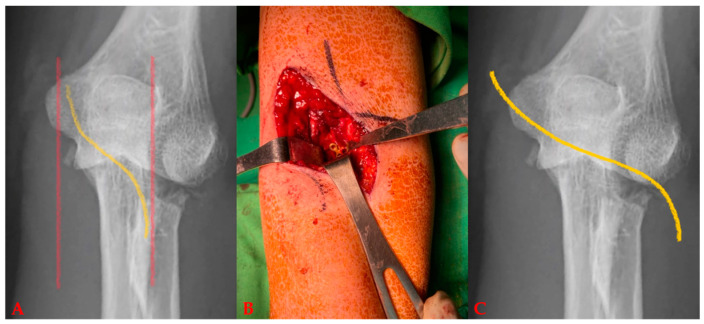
The fluoroscopy-guided ulnar anteromedial (FGUAM) approach: (A) under a plain radiograph, the curvilinear incision (yellow line) starts from the top of the ulnar border (red line) of the olecranon process, extending distally to the top of the bicipital tuberosity, (B) anterior fixation with a plate, (C) the original AM approach has the S-shaped incision (yellow line) from the ulnar side to the radial side in the antecubital fossa.
